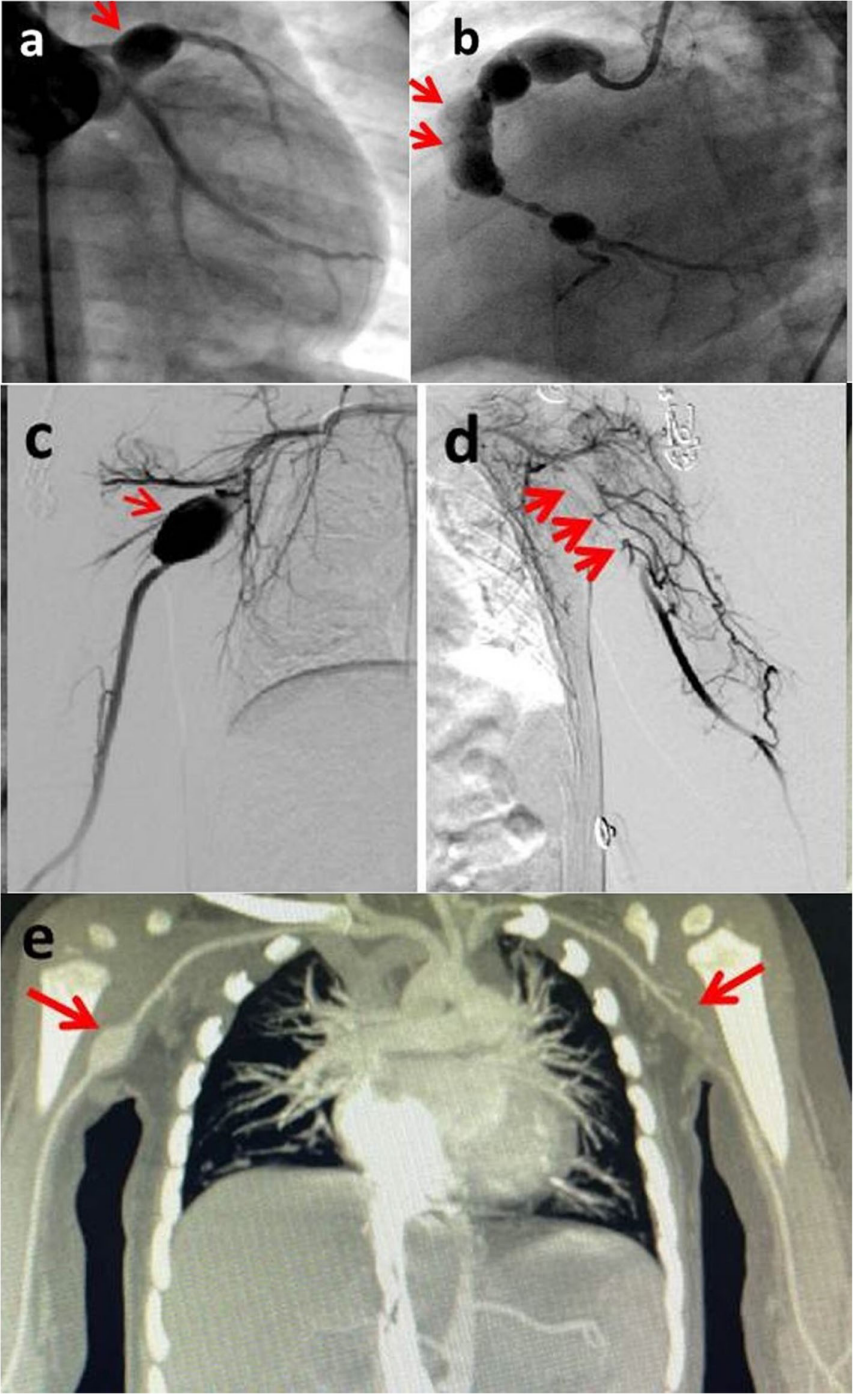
Coronary angiography shows: a A 6.0*8.5 mm aneurysm (arrow) at the bifurcation of LAD and circumflex branch. b Beaded aneurysms in the RCA from proximal branch to the bifurcation of the posterior descending coronary artery. An incomplete thrombus can be seen in the third aneurysm (arrows). Peripheral arteriography shows: c Right giant AAA (arrow) with normal blood flow. d Left giant AAA which has been completely occluded (arrows) with fine collateral vessels supplying blood to the distal brachial artery. e CT angiogram at 3-month follow-up shows more blood flow to the left brachial artery distal to the occluded AAA compared to the images 3 months prior (d)
